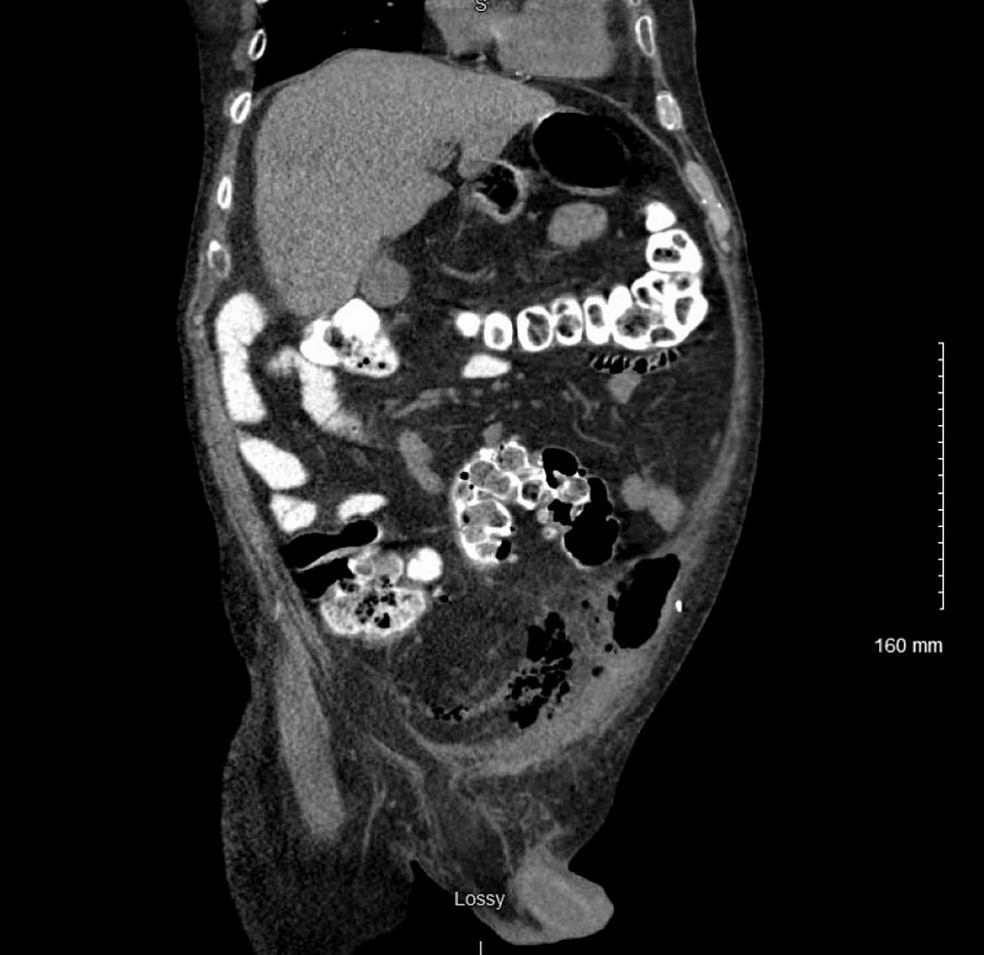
CT abdomen and pelvis with oral and rectal contrast demonstrating no contrast extravasation but air bubbles arising from a necrotic lymph node
